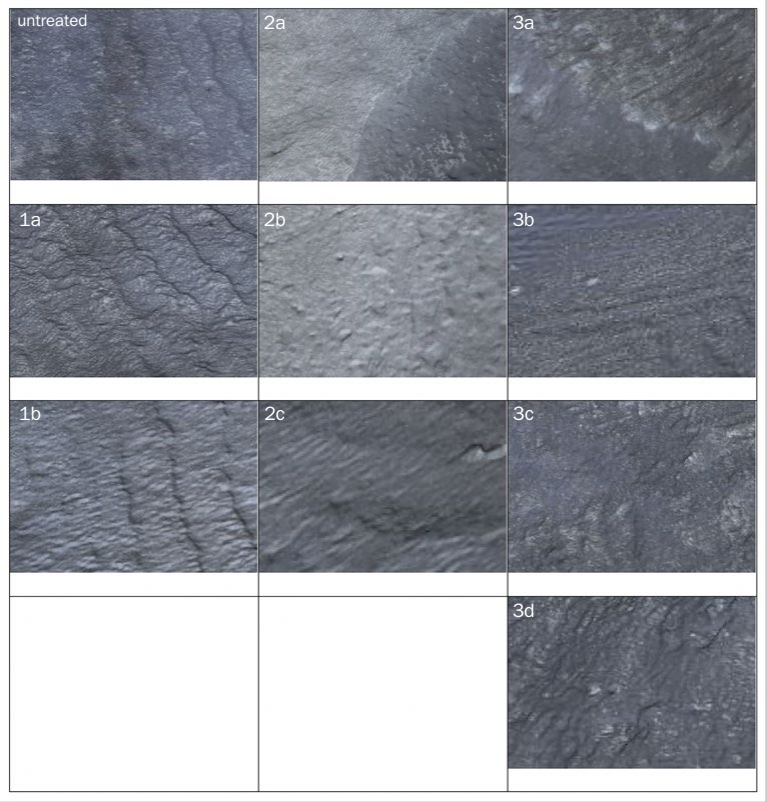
Enamel at 20X magnification: (1a) air polishing only or (1b) with rubber-cup polishing; (2a) ultrasonic scaling only or (2b) with air polishing or (2c) with rubber-cup polishing; (3a) hand scaling only or (3b) with air polishing or (3c) with rubber-cup polishing or (3d) with both.
